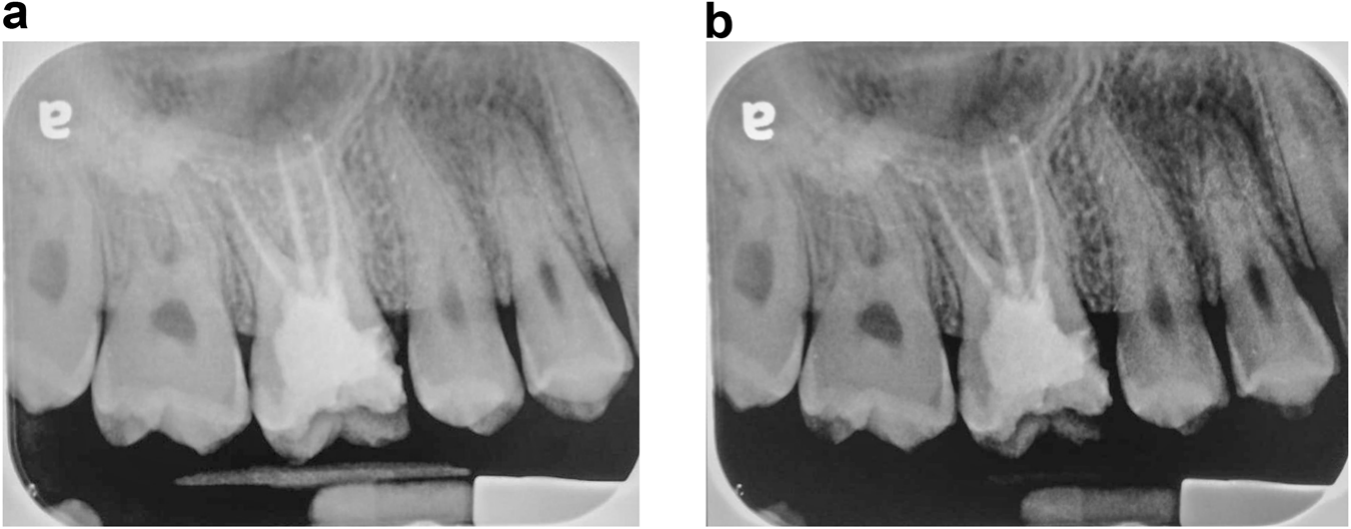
A periapicl x-ray of tooth #16, in both commercial screen and a diagnostic screen, photographed using a digital camera. a, b Tooth #16: The PDL of the buccal roots was identified as “widened” when using DS and not “not widened” when using CS (a = CS, b = DS).
